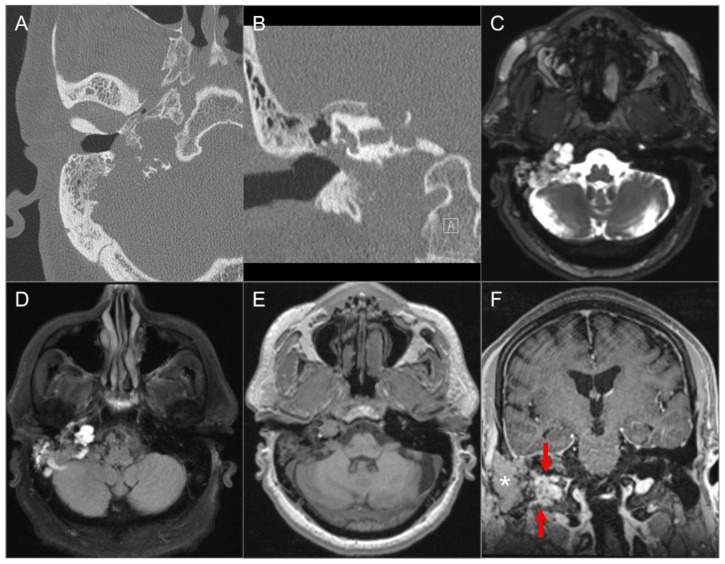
Endolymphatic sac tumor. (A) Axial and (B) coronal CT images show a lytic lesion with a moth-eaten, permeative appearance in the right retro-labyrinthine petrous temporal bone extending to the middle ear cavity, mastoid region, and jugular fossa. (C) Axial fat-suppressed T2W, (D) axial FLAIR, and (E) axial noncontrast T1W images demonstrate a complex solid and cystic lesion with mixed signal intensity, likely reflecting hemorrhagic and proteinaceous contents. (F) Postoperative coronal postcontrast T1-weighted image shows heterogeneous enhancement in the residual solid component of the lesion (red arrows). The more lateral tissue corresponds to a fat graft (asterisk).
